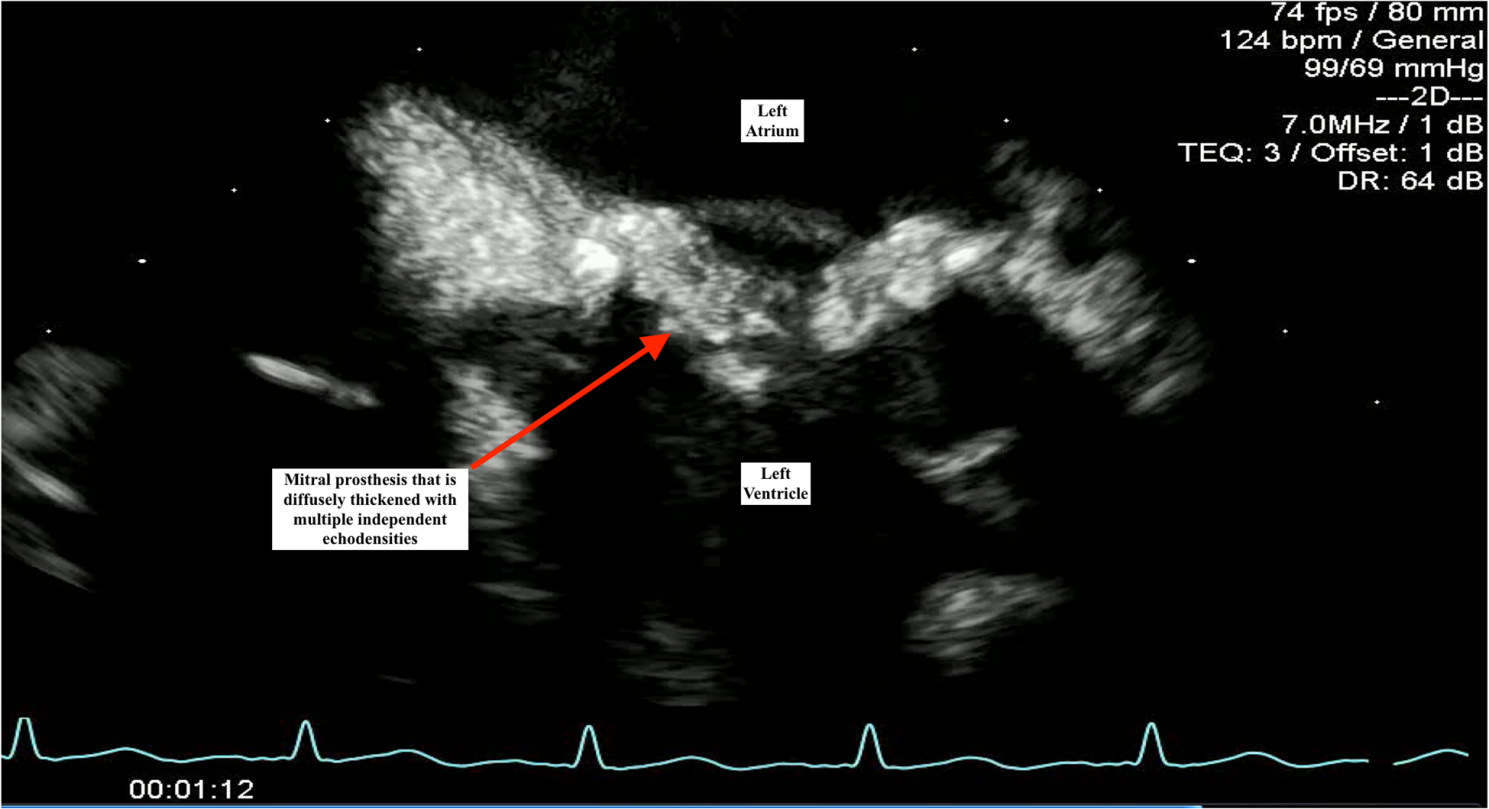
Transesophageal echocardiography showing multiple independent echodensities on the leaflets with significant soft tissue infiltration of the valve itself
